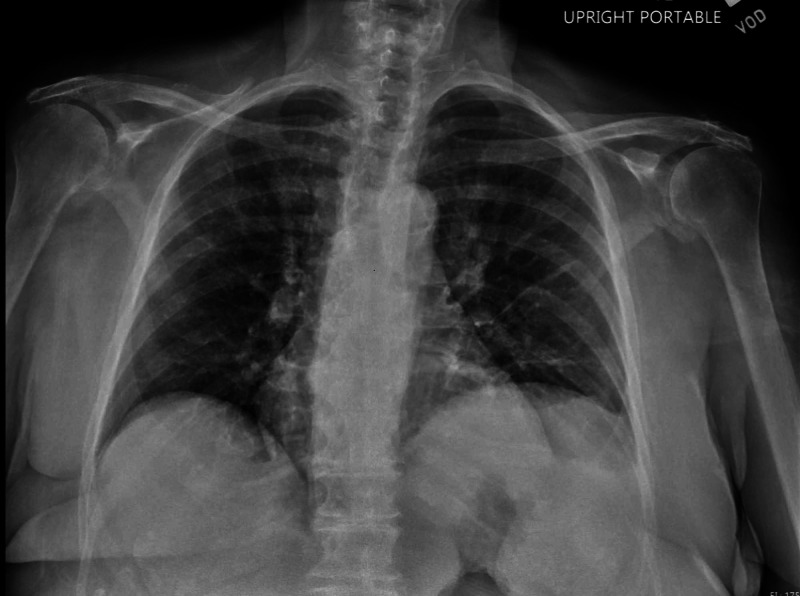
Chest X-Ray (anterior-posterior view) No cardiopulmonary pathology.
